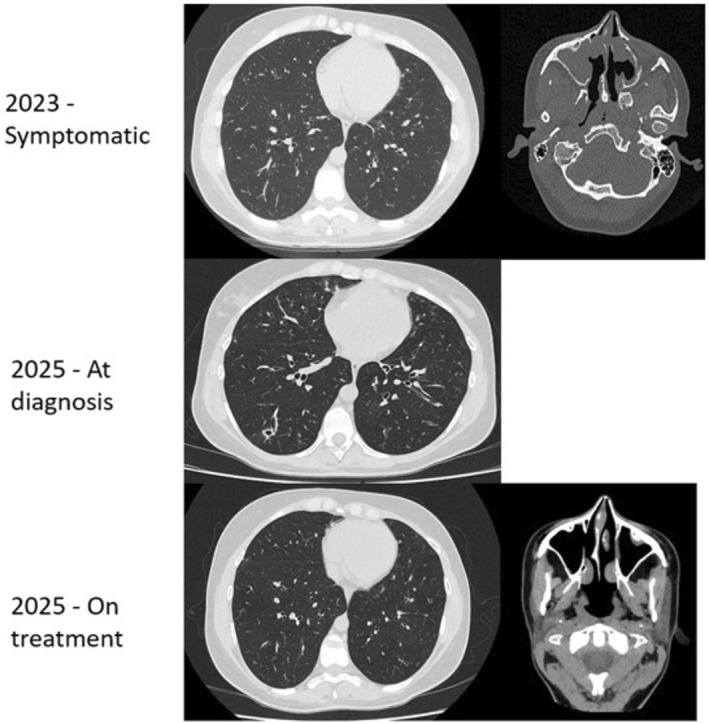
CT sinus and chest imaging prior, at time of *M. abscessus* diagnosis and once established on treatment.
